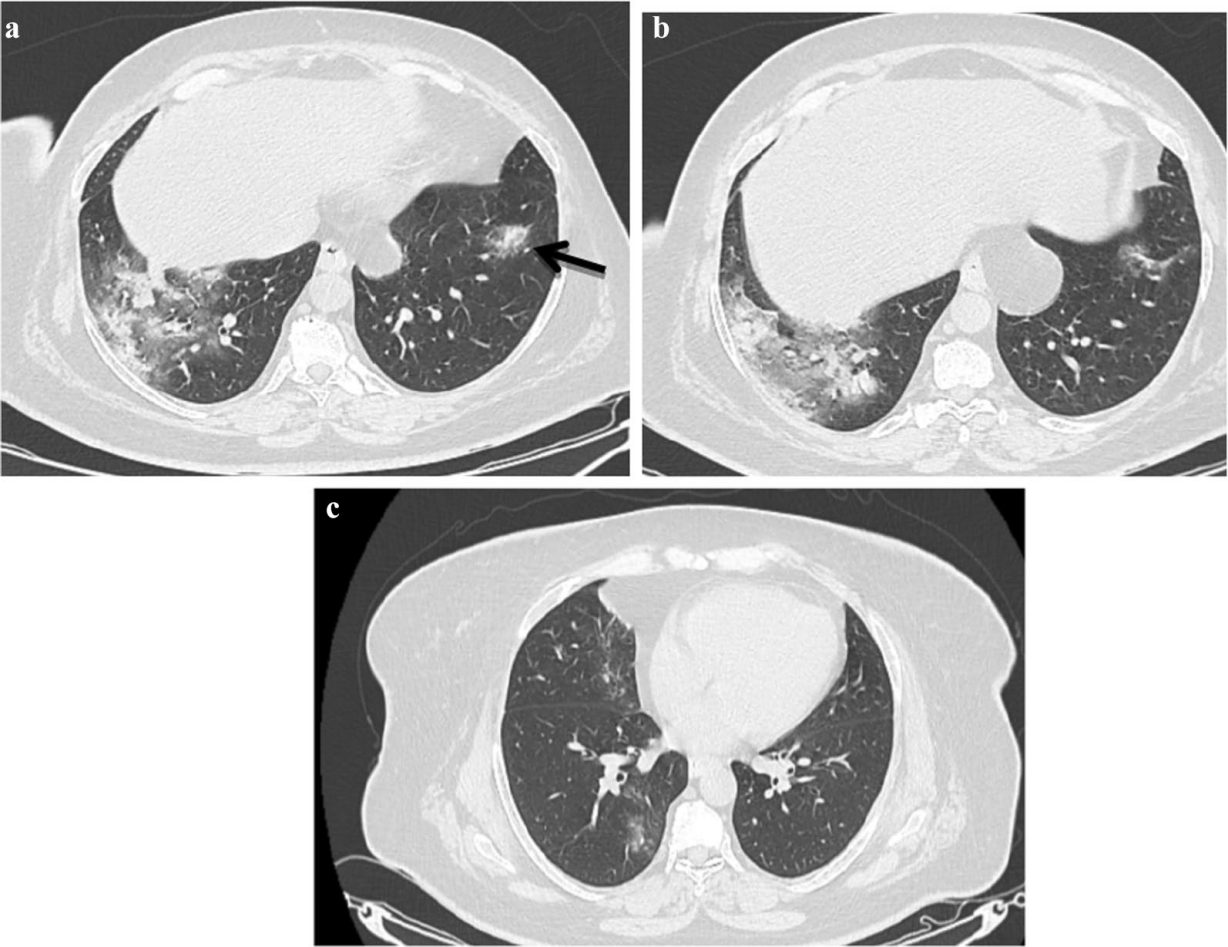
A 47-year-old female known history of rheumatoid arthritis presented with anosmia, loss of taste, fever, diarrhea and myalgia at May 2020 and positive PCR for COVID 19 infection, a and b noncontrast CT axial cuts shows bilateral patchy subpleural ground glass opacities and consolidations with left lower lobe nodule with ground glass halo sign noted. The patient diagnosed as COVID 19 infection with CT score severity: 16. Second infection dated June 2021 the patient presented with anosmia, loss of taste, fever, diarrhea and myalgia with positive PCR test for COVID 19 infection. c noncontrast CT chest images show bilateral patchy subpleural ground glass opacities. The patient diagnosed as COVID 19 re-infection with CT severity score: 5
